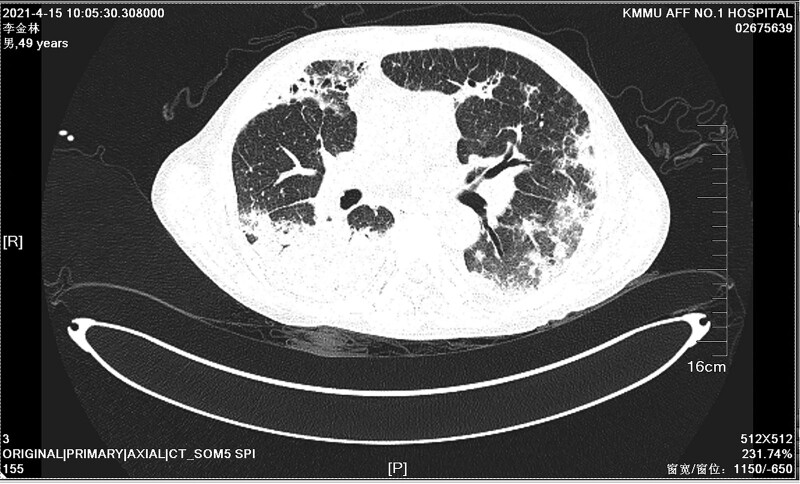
Chest CT on April 15, 2021 showed that the large flaky consolidation of the upper and lower lobes of the right lung was absorbed more than the previous part, and the bronchial air sign was seen inside, and the size of the lungs was multiple. The nodules vary from one to the other, some of them are smaller than before, the upper lobules of the lungs are thickened, and the exudation is absorbed more than before, and the mediastinum still has no clear enlargement of the lymph nodes. CT = computed tomography.
